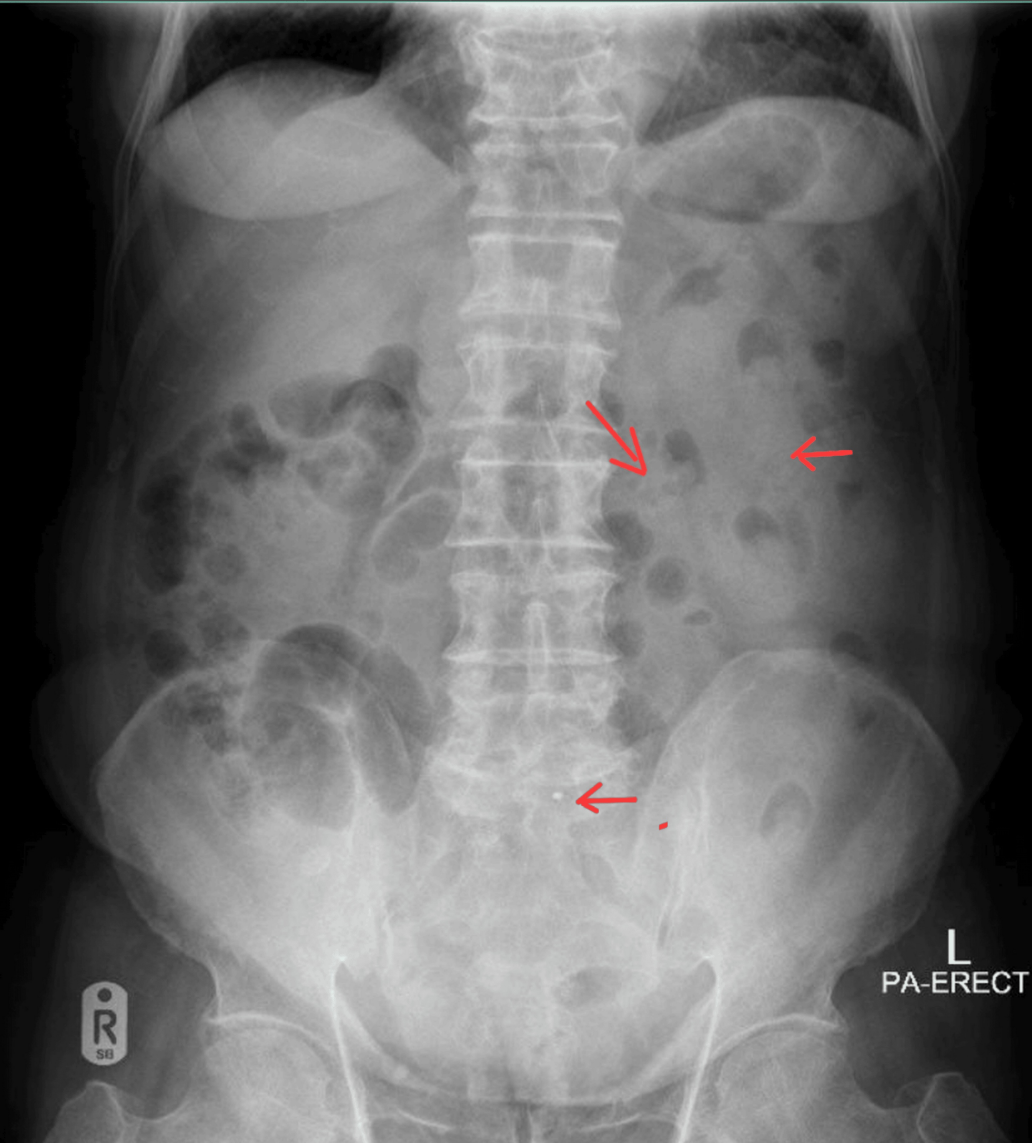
Abdominal X-Ray (XR) Fecal loading in the colon (red arrows).
